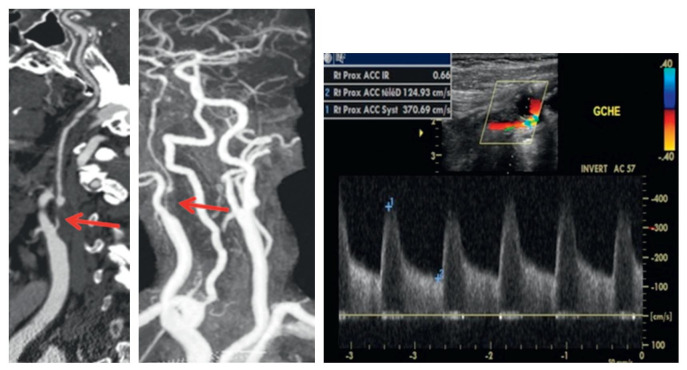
Angio-scanner (a) and MRA (b) of the supra-aortic trunks, carotid Doppler (c). Tight right carotid stenosis (from Vignal-Clermont C, Tilikete C, Milea D. Neuro-ophtalmologie. 2e edition, 2016, Elsevier)
